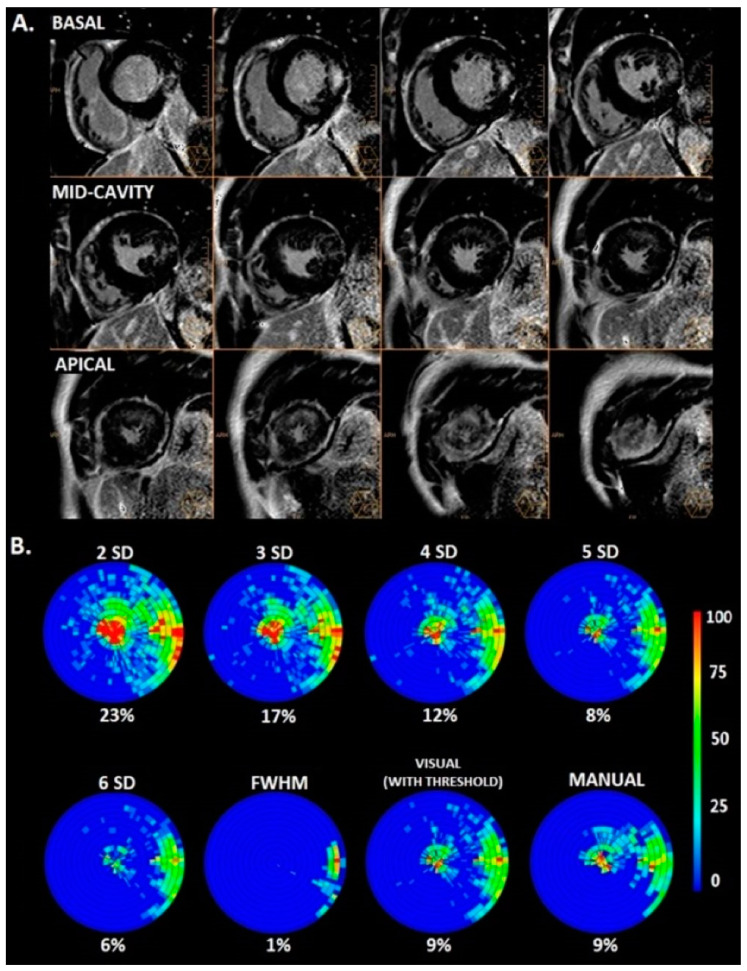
Examples of differences in late gadolinium enhancement quantified by different methods used in cardiac magnetic resonance imaging in patients with Fabry disease. Legend: (A) Short-axis cardiac magnetic resonance images with late gadolinium enhancement in a patient with Fabry disease; (B) bullseye graphical representation of late gadolinium enhancement quantified by different methods used in the same patient with Fabry disease. The color scale represents the late gadolinium enhanced volume in the left ventricle (percentage). Abbreviations: 2SD, 3SD, 4SD, 5SD, 6SD—grayscale thresholds with 2, 3, 4, 5 or 6 (respectively) standard deviations above the mean signal intensity for the remote myocardial tissue; FWHM, full width at half maximum method.
